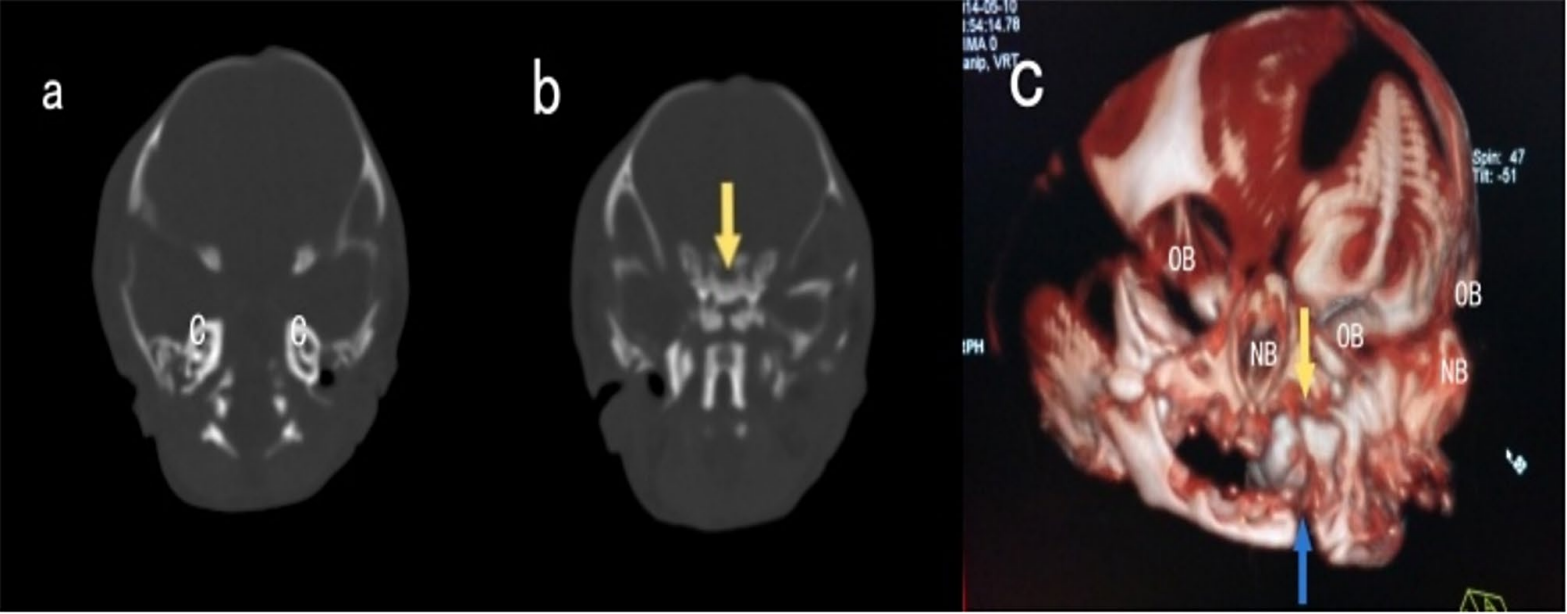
Postnatal CT images of this case (a) show two fully developed cochleae (C: Cochlea). (b) shows fusion of the two sphenoid bones, as indicated by the arrow in the middle (Sph: Sphenoid Bone). (c) CT three-dimensional imaging reveals the fusion of two nasal bones, two fused maxillae (indicated by the arrows) and two fused mandibles (indicated by the blue arrows), as well as three orbital bones (OB: Orbital Bones; NB: Nasal Bone)
